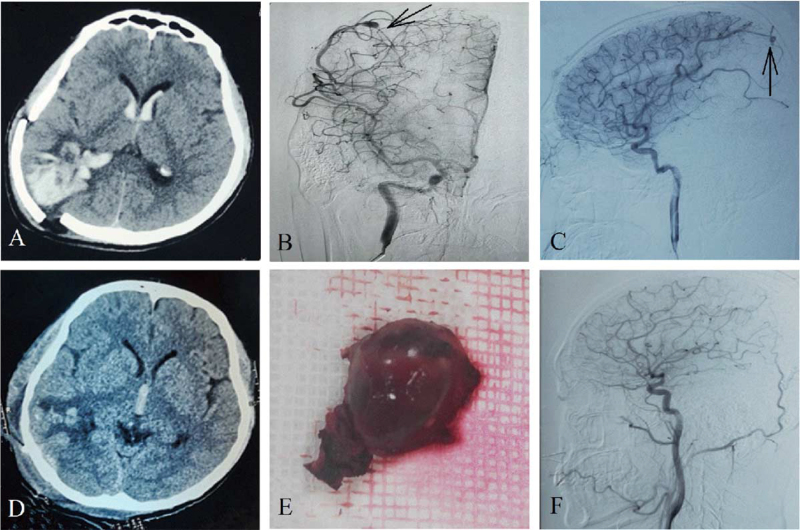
Patient's imaging data during the second ICH. (A) The second head CT showed right parietal hemorrhage into the ventricular system. (B, C) The second head DSA (B: anteroposterior view; C: lateral view; black arrow) showed a cystic aneurysm in its original position. Thus, aneurysm recurrence was considered. (D) Aneurysm resection and removal of ICH were performed during the second operation. The head CT showed that the hematoma was completely removed. (E) Removed aneurysm tissue. (F) In the third head DSA (lateral view) no aneurysms were observed, and there was nothing unusual regarding the course of the intracranial arteries. CT = computer tomography, DSA = digital subtraction angiography, ICH = intracerebral hemorrhage.
